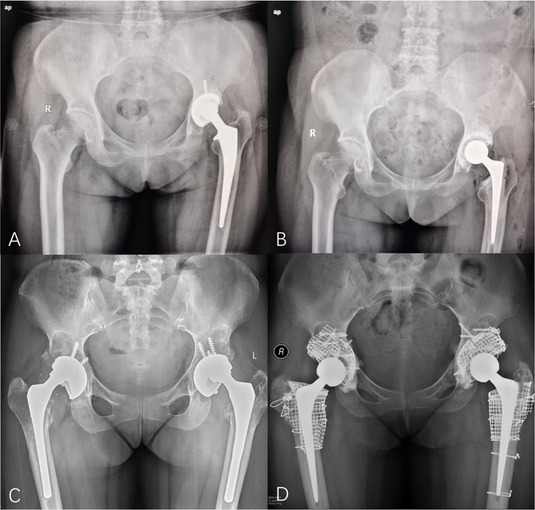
A, B Pre-op and Post-op X-ray of one case with Paprosky Type I defect; C, D Pre-op and Post-op X-ray of one case with bilateral Paprosky Type II defect
